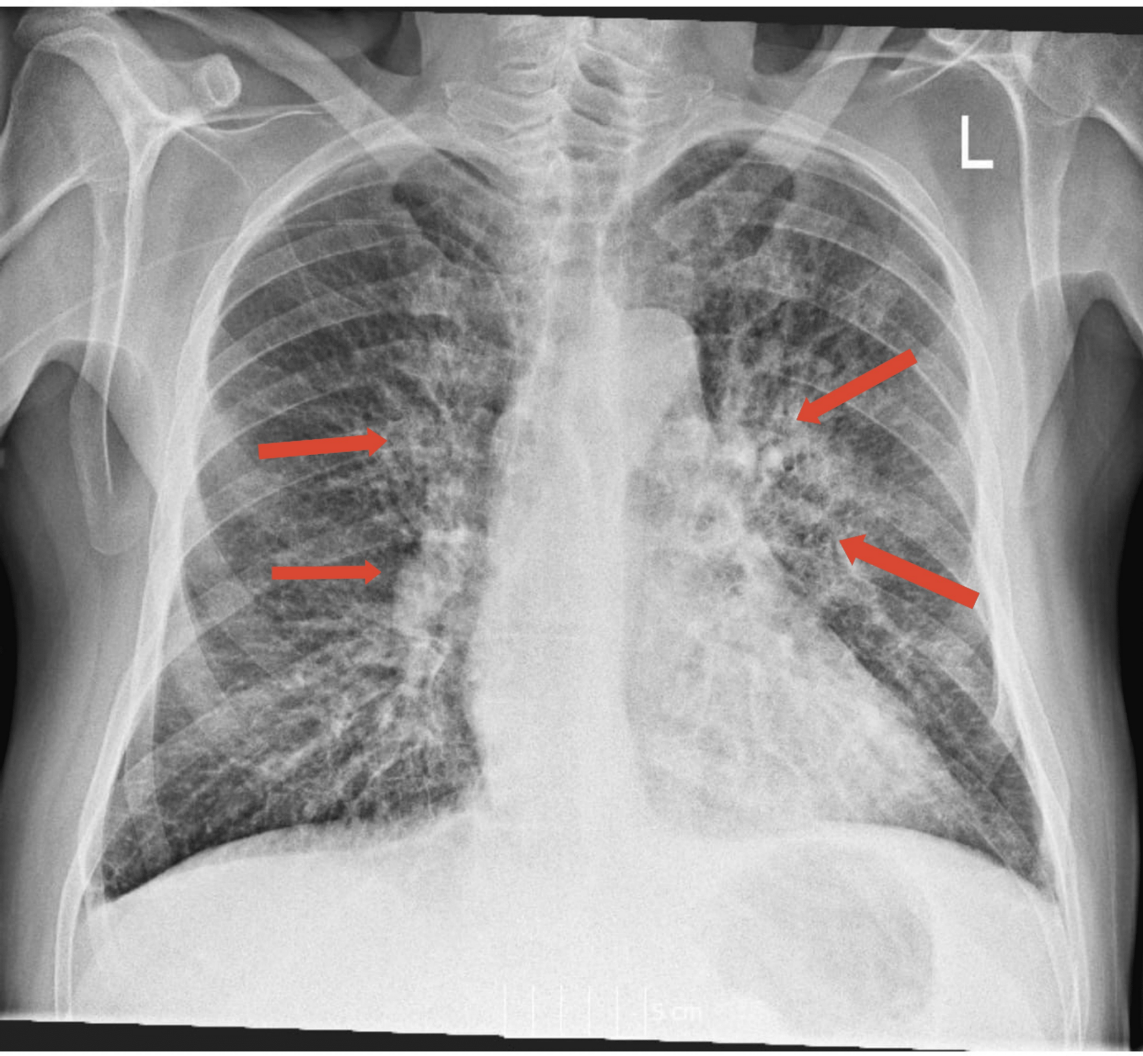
Chest X-ray taken at the time of hospital presentation. Chest X-ray taken at the time of hospital presentation, showing new bilateral diffuse perihilar inflammatory changes (indicated by arrows).
